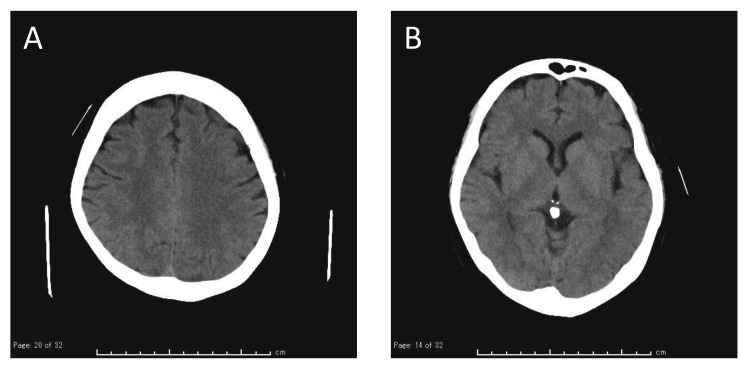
(A-B) Brain CT of Case 2 showing no acute lesions consistent with vasculitis
